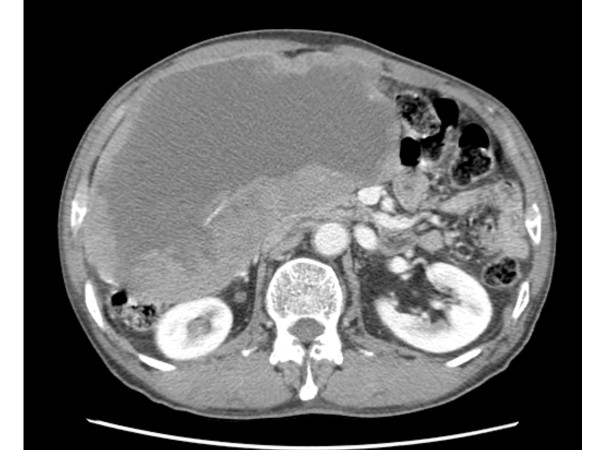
Initial CT scan showed a 20 × 19 cm intraabdominal mass with central necrosis in the infra-hepatic space and tumor invasion into the peritoneum.
